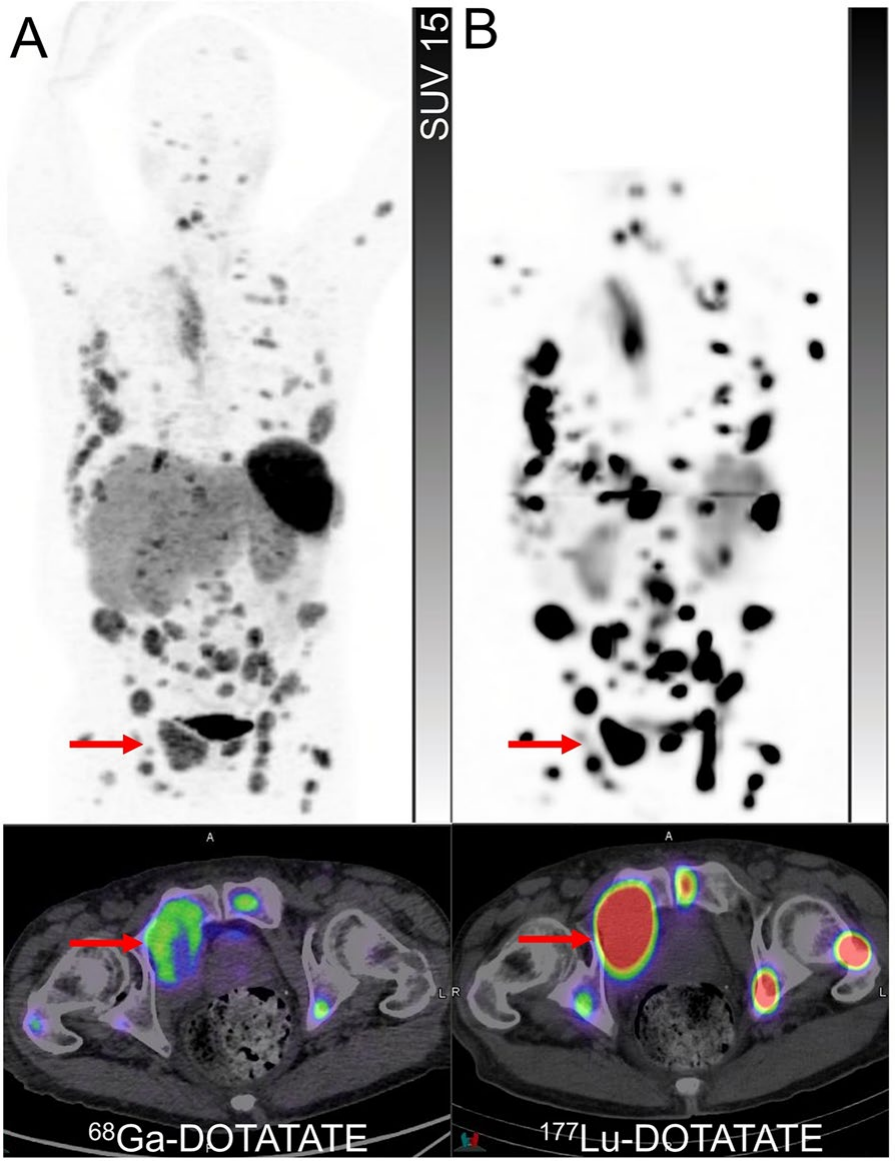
A patient with small cell lung cancer with progression after platinum-based chemotherapy and immune checkpoint monoclonal antibodies. ^68^Ga-DOTATATE PET and PET/CT (A) showed extensive moderately avid (Krenning score 3) osteolytic bone metastases (arrows). In the absence of other treatment options, the patient underwent ^177^Lu-DOTATATE, and post-treatment SPECT and SPECT/CT at 24 h (B) showed very high tracer retention (Krenning score 4), much higher than expected from baseline ^68^Ga-DOTATATE PET, in all sites of disease
